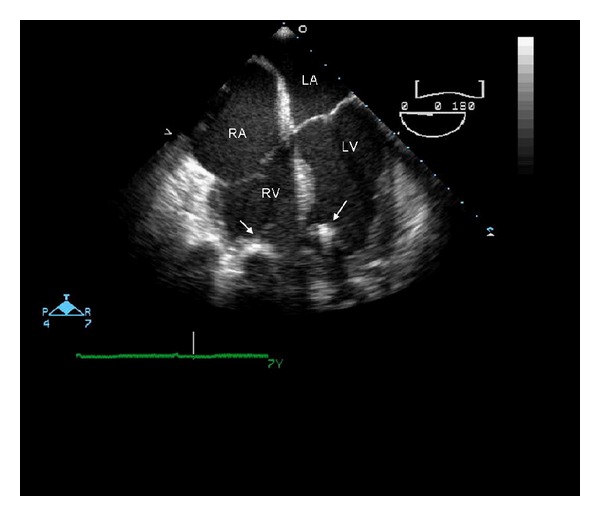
Transoesophageal echocardiogram 4 chamber view: biventricular assist device inlet cannulas in the left and right ventricles are shown (arrow). RA: right atrium, LA: left atrium, RV: right ventricle and LV: left ventricle.
